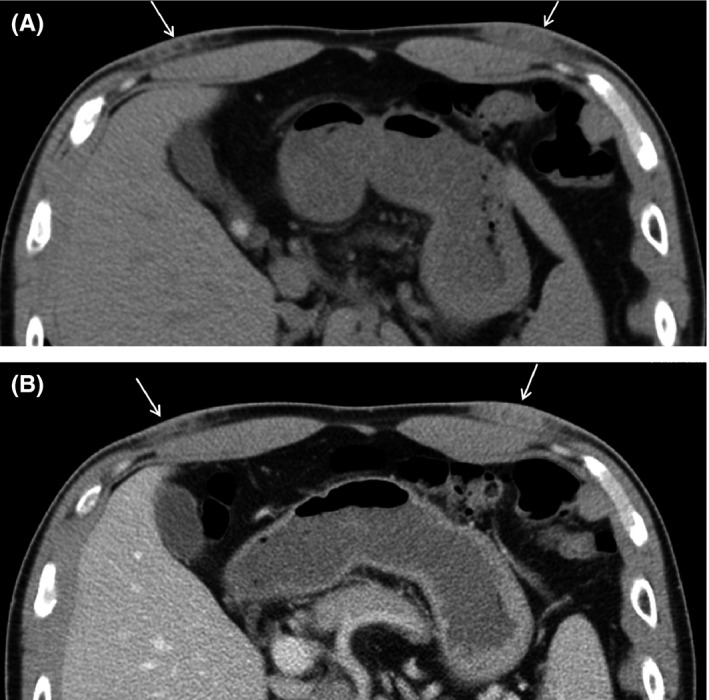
Plain CT (A) and contrast‐enhanced CT (B) revealed soft tissue density mass lesion with ill‐defined margin in subcutaneous region of bilateral anterior chest (arrows)
